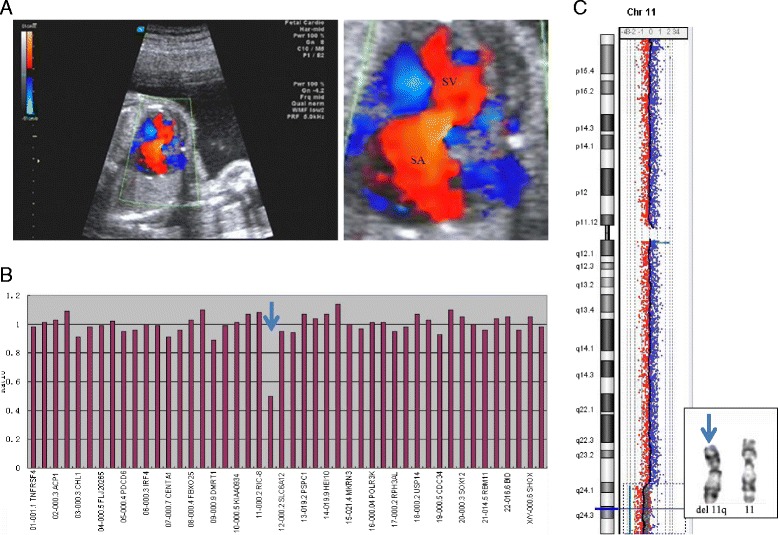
Ultrasound findings and chromosomal abnormality characterized in case 2. A. A four chamber view shows single ventricle (SV), single atrium (SA) and common atrioventricular valve. A color Doppler flow image shows the bloodstream of atrioventricular valve was flowed from SV to SA. B. MLPA shows an 11q deletion (arrow). C. aCGH reveals a 13.062 Mb deletion of 11q24.1q25 (inset, arrow points to the distal deleted chromosome 11).
